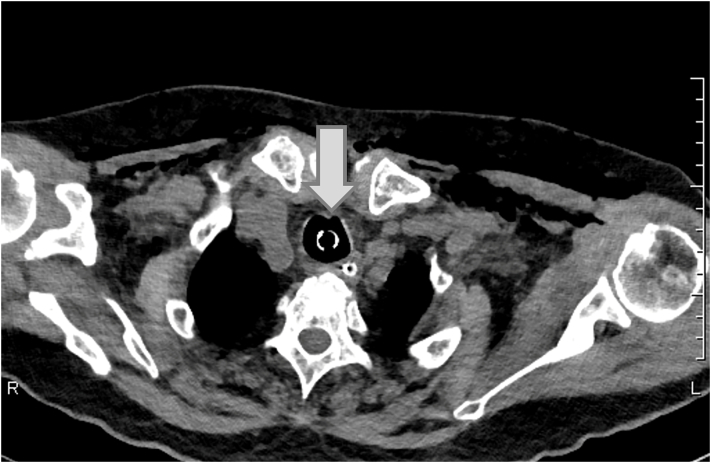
Plain computed tomography on day 4 showing anterior wall irregularity of the trachea was undetectable (arrow).
